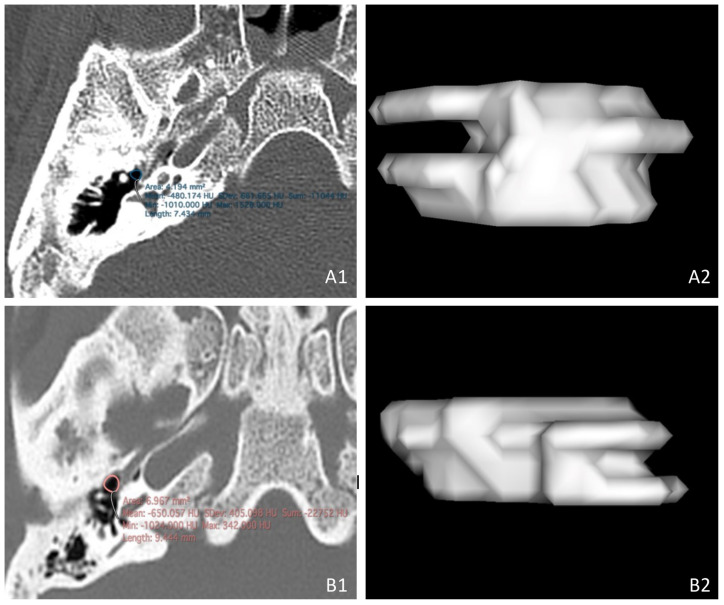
Axial HRCT image showing the measurement of the right STR in a sclerotic mastoid (A1); corresponding 3D volumetric reconstruction of the STR, with a measured volume of 3.9 mm^3^ (A2). Axial HRCT image showing the measurement of the right STR in a diploic mastoid (B1); corresponding 3D volumetric reconstruction of the STR, with a measured volume of 5.4 mm^3^ (B2).
